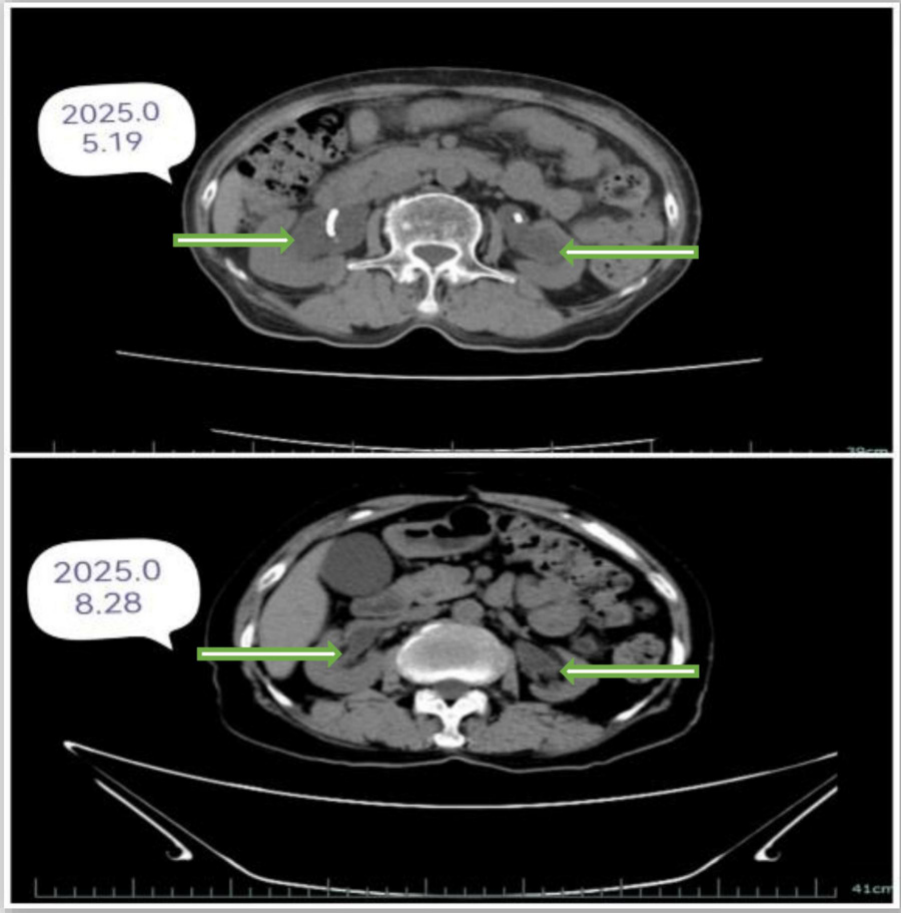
CT indicates improvement in hydronephrosis 3 months postoperatively.
